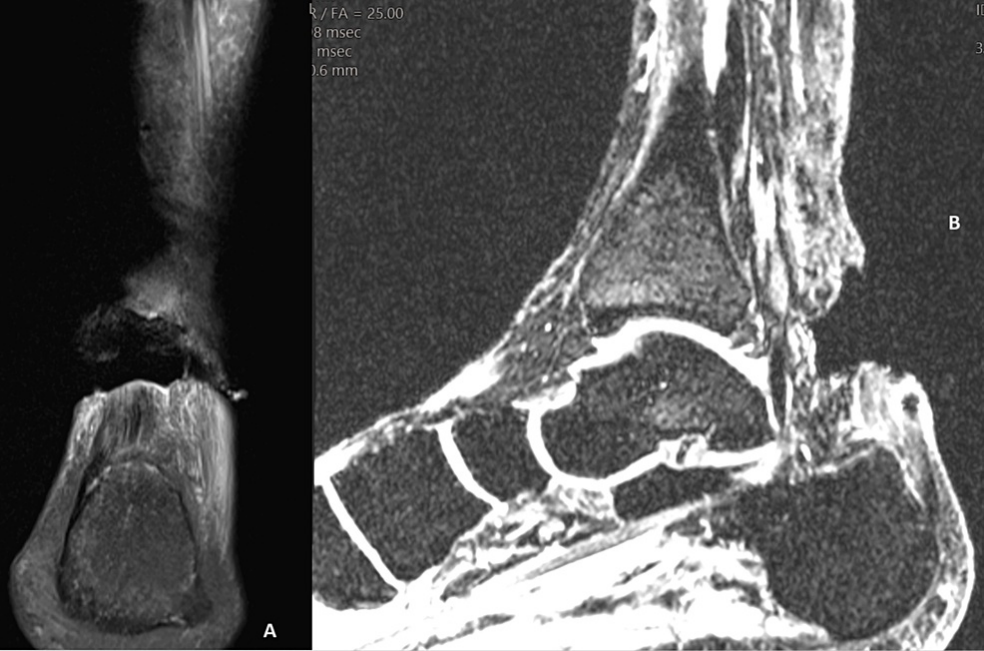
MRI images of the Achilles tendon rupture
